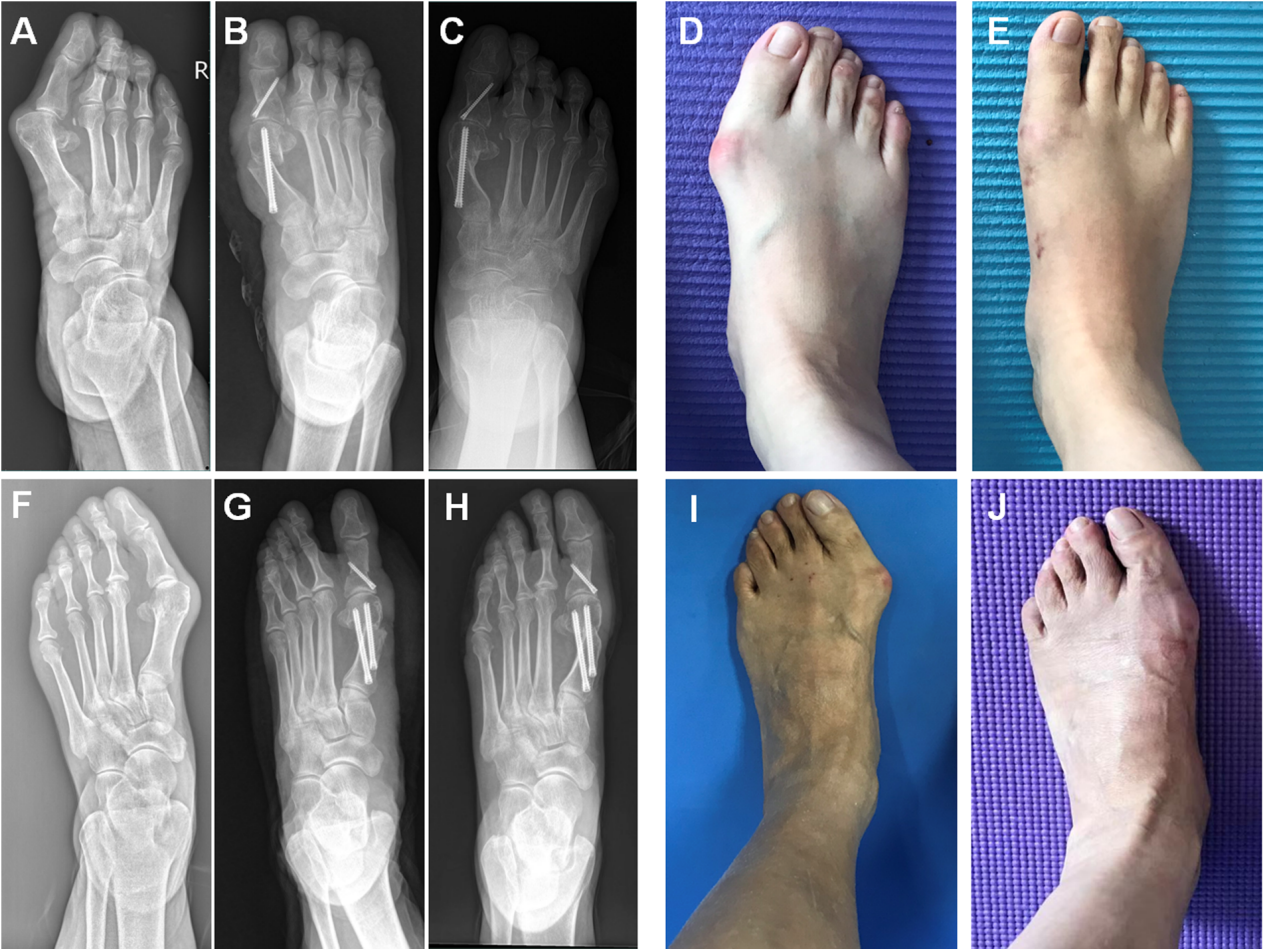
(A,F) Radiography showed moderate hallux valgus deformity. (D,I) Corresponding clinical photo. Radiography showed the correction of hallux valgus deformity after the single-screw fixation MICA (B) and the dual-screw fixation MICA (G). (C,H) 24 months after the operation and corresponding clinical photo (E,J).
